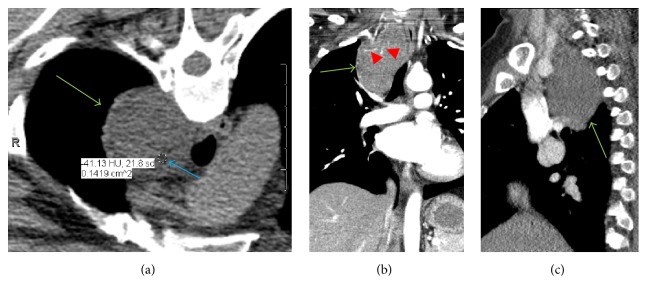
Contrast enhanced axial CT (a) with coronal (b) and sagittal (c) CT reconstructions of the chest demonstrating a well circumscribed, heterogeneously enhancing mass (green arrows) with areas of fat attenuation (blue arrow pointing to ROI in (a)) and a few mildly prominent internal vessels (red arrow heads in (b)) within the superior, posterior right mediastinum.
